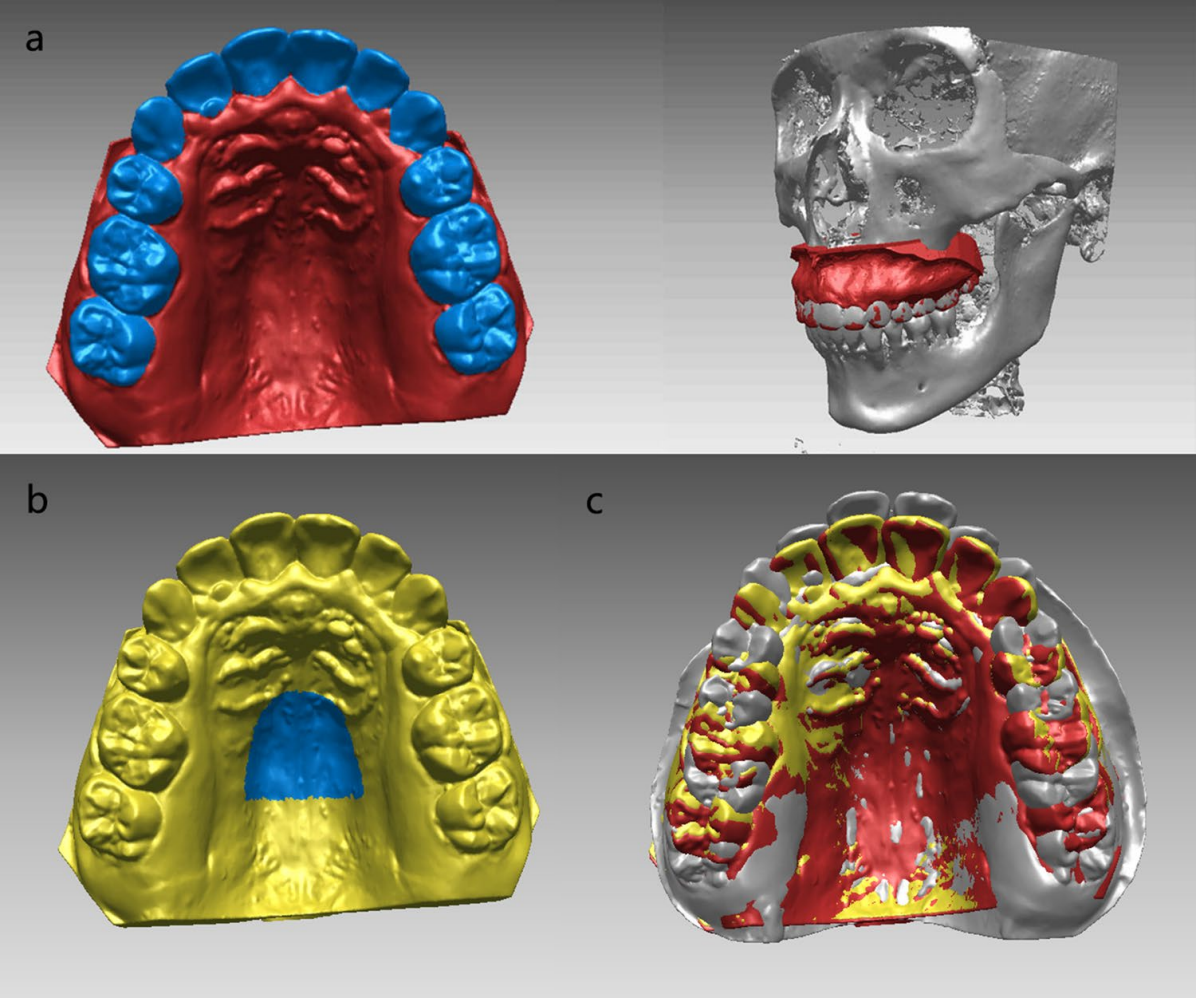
(a) Dental crown areas (blue area) as a reference for CBCT-MDM superimposition on T2 model (red); (b) palatal vault region (blue area) as a reference for superimposition of T1 (grey) and T2′ (yellow) models; (c) superimposed T1 (grey), T2 (red) and T2′ (yellow) models.
